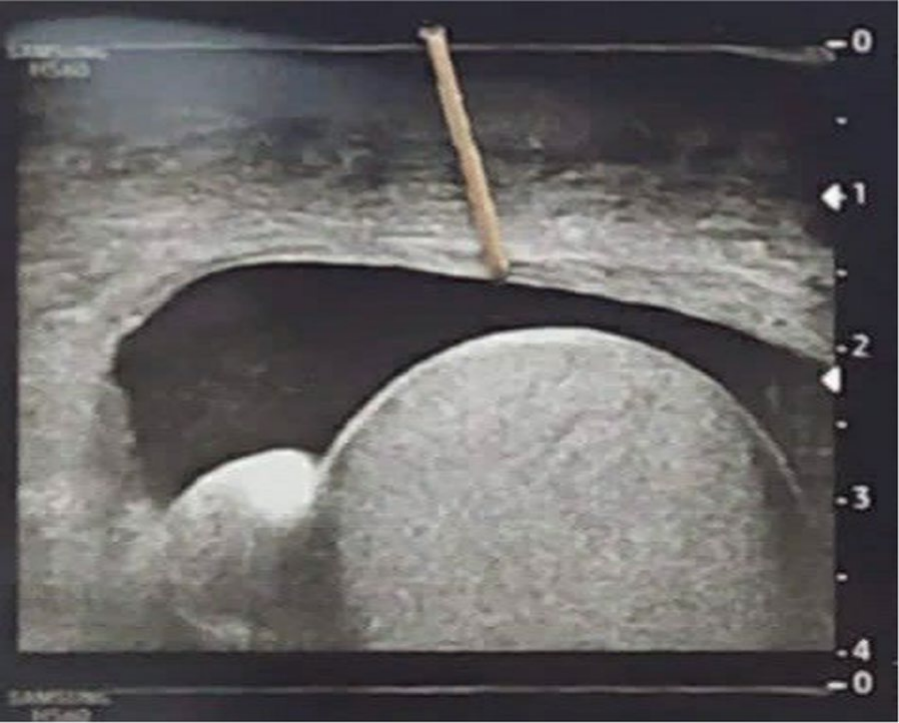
Ultrasound of the testis; small right-sided hematoma. Normal size and vascularity of right testis
